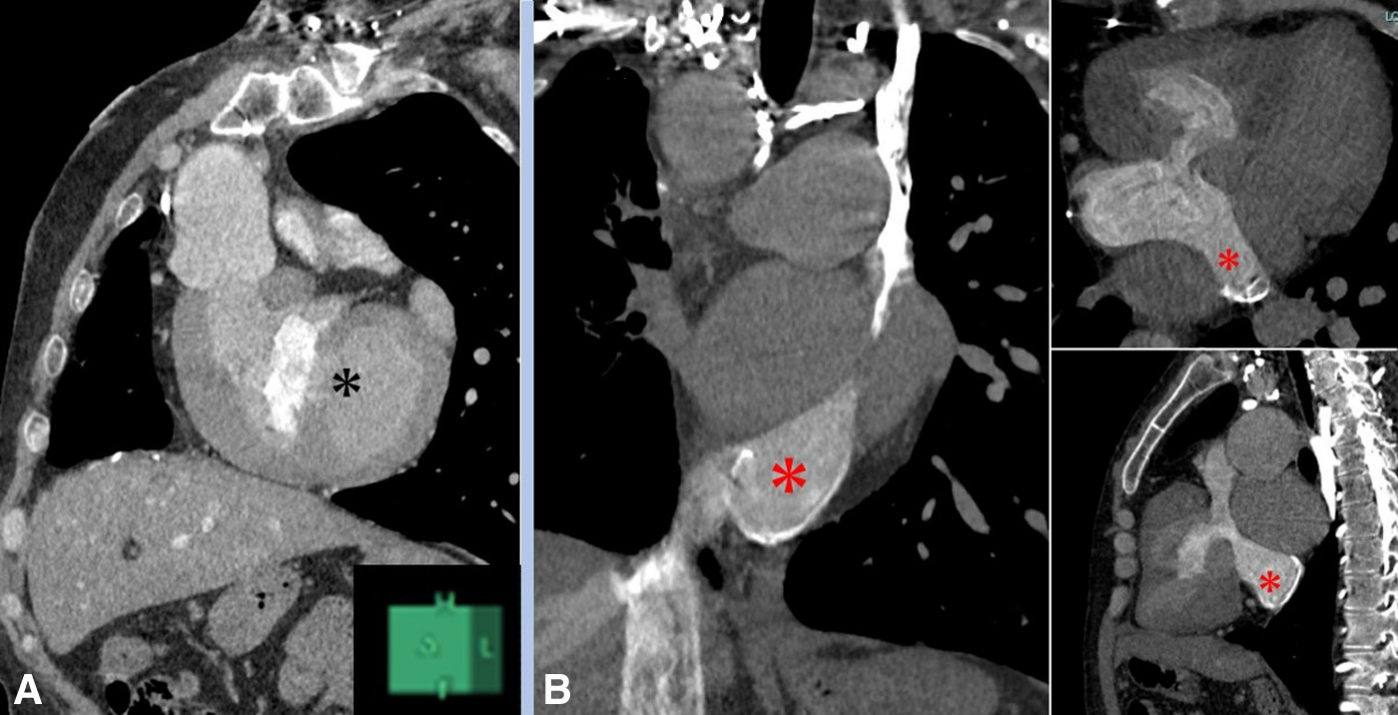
Preoperative contrast computed tomography images. A, Remote ventricular septal defect (*black asterisk*). B, Dilated coronary sinus (*red asterisk*) in coronal, horizontal, and sagittal planes.
